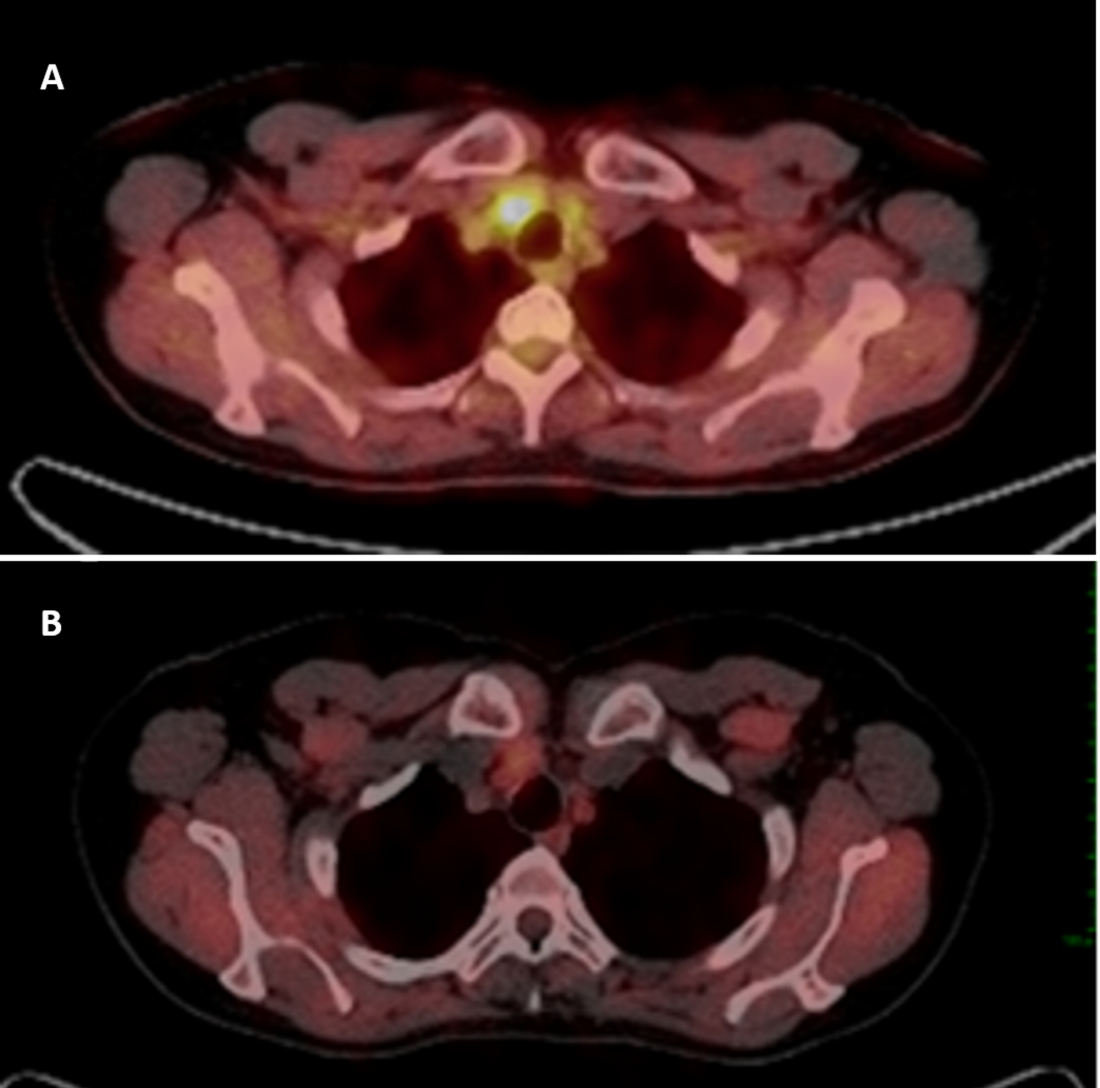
PET-CT A. PET-CT showing hypermetabolic right paratracheal and hilar lymphadenopathy, stable in size but with 20% reduction of the metabolic activity compared with previous study (image not available), SUVmax 3.8 (Date: Feb/2018). B. PET-CT showing parahilar lymphadenopathy with significant decrease of the activity compared to previous images, without significant changes in size, SUVmax 2.4 (Date: Jul/2019). Abbreviations: PET-CT, positron-emission computed tomography; SUVmax, maximum standardized uptake value.
